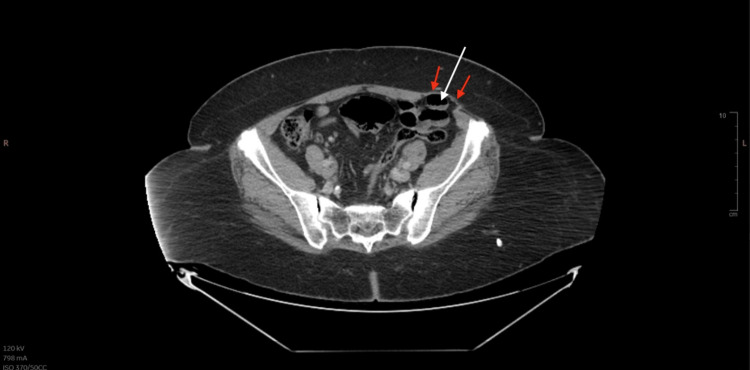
CT of the abdomen and pelvis post-contrast displaying a left spigelian hernia with small bowel content (white arrow). Also seen is the intact aponeurosis of the external abdominal oblique (red arrows).
